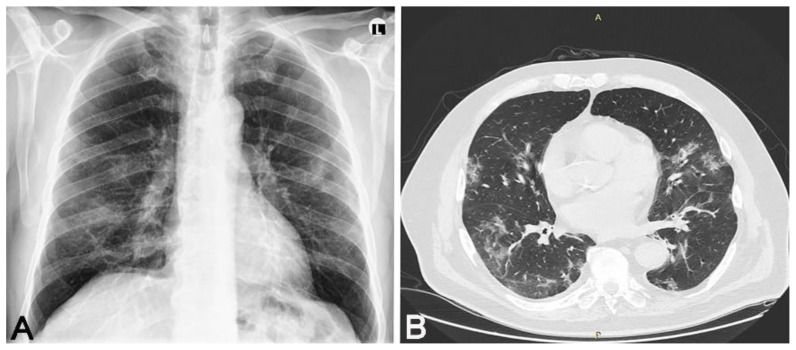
Thirty-eight-year-old male patient without any chronic diseases was admitted to the emergency department as a SARS-CoV-2 positive with high fever, cough, dyspnea and fatigue. Chest X-ray showed (A) bilaterally gentle inhomogeneous shading initial infiltrative changes. After 9 days from the onset of infection, dyspnea worsened, chest MSCT showed (B) areas of enhanced attenuation by the type of ground glass, and patchy consolidations are seen on both sides. The patient developed bilateral pneumonia. Legend: L—left side, small A letter on panel B: A—anterior, P—posterior.
